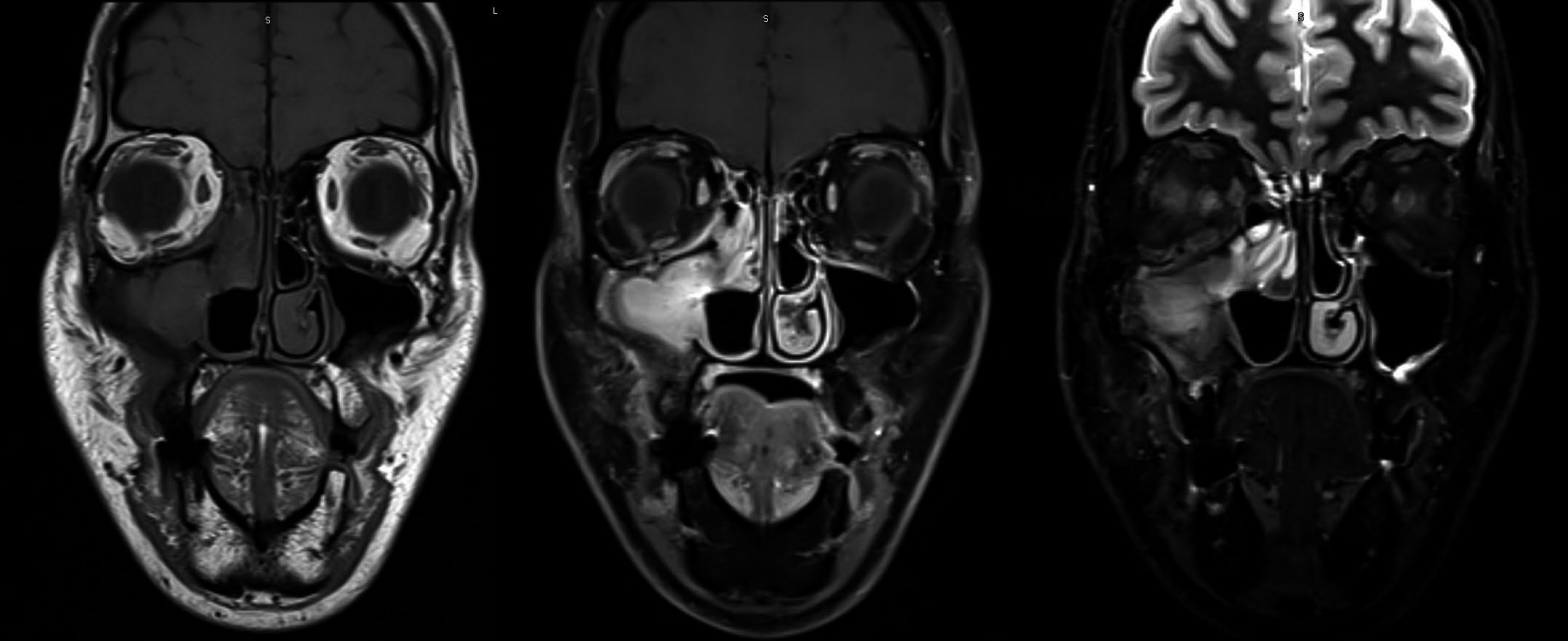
T1, T1 with and T2 with contrast MRI showing enhancing mass lesion in the right maxillary sinus
